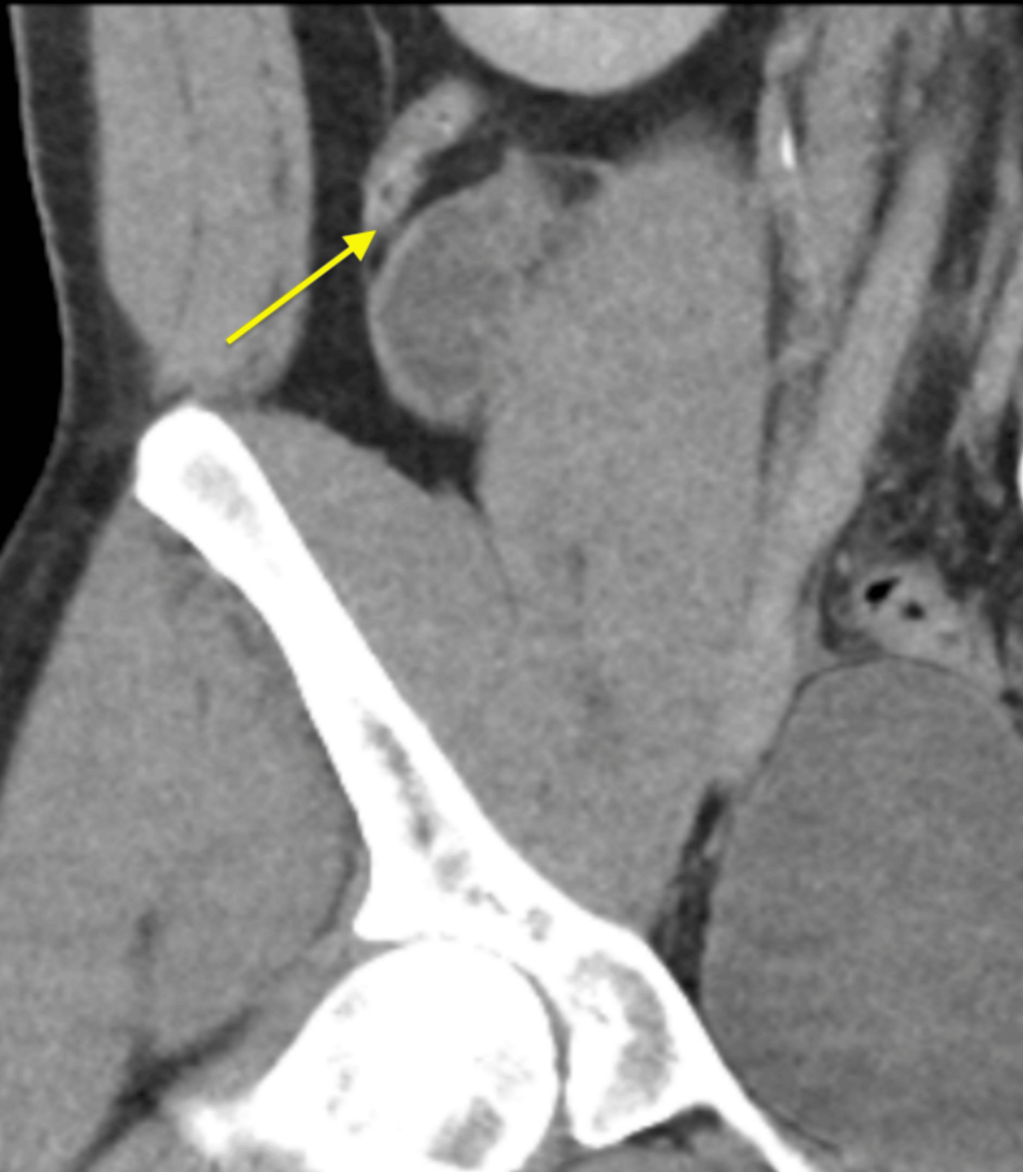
CT abdomen/pelvis with contrast showing a mildly thickened appendix measuring 8 mm without signs of perforation, similar to the imaging without contrast (yellow arrow).
